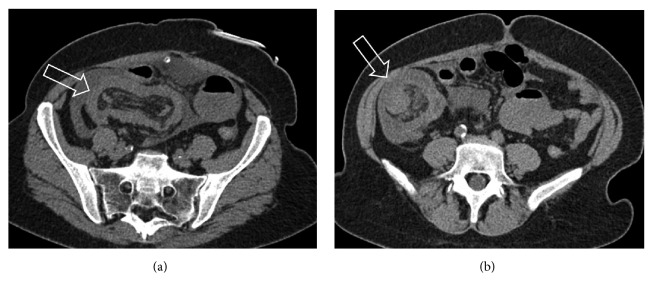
CT scan images showing ileocecal intussusception (arrows) from a 2 cm neuroendocrine tumor arising from the terminal ileum.
